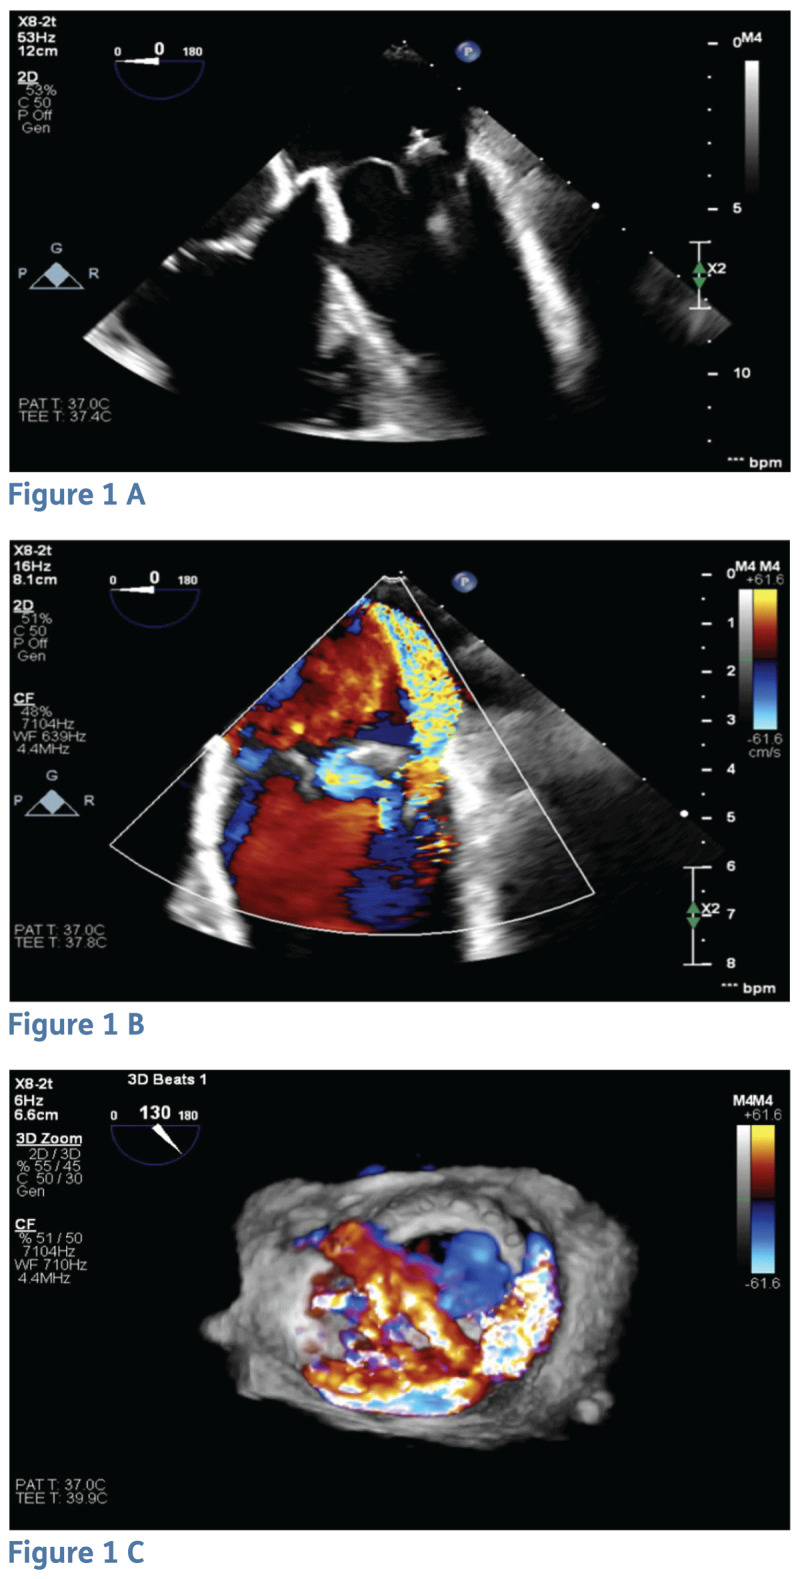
Transesophageal echocardiogram view of bioprosthetic mitral valve showing (A) valvular dehiscence, (B) color Doppler of severe paravalvular leak, and (C) 3-dimensional image.
